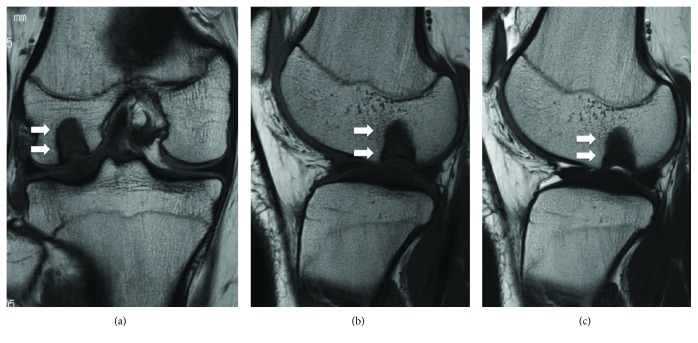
(a) Coronal T1-weighted MR image reveals the lesion growing larger. (b, c) Sagittal T1 and T2-weighted images show a low-signal-intensity lesion (arrow).
